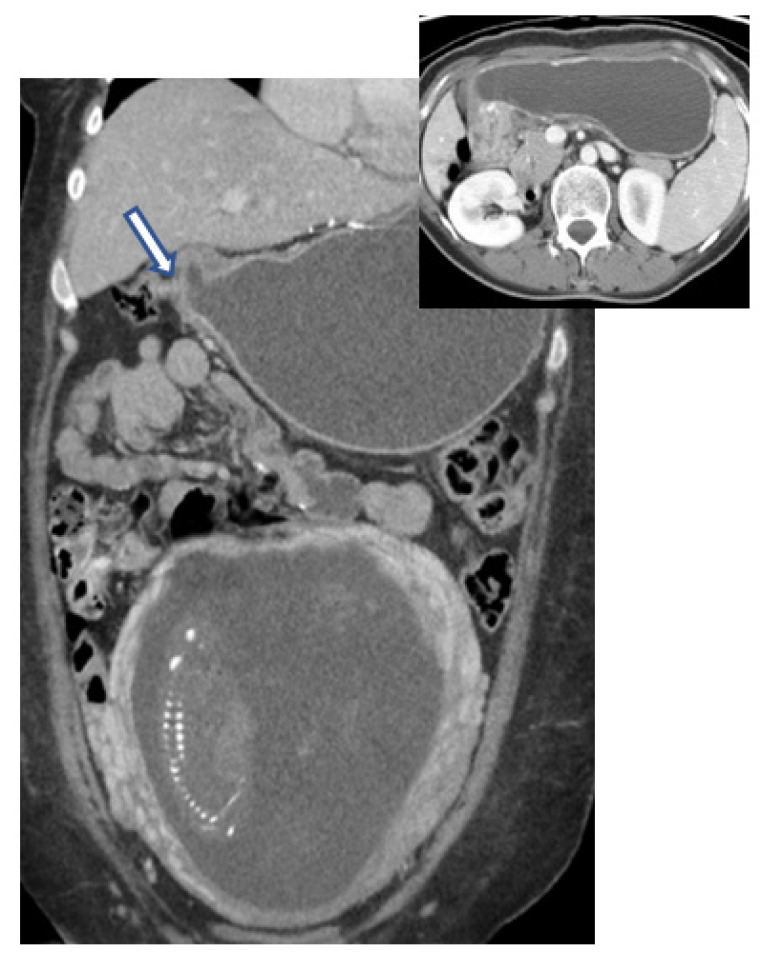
Computed tomography imaging of a pregnant woman (gestational age 16 weeks) with pyloric obstruction (arrow) caused by gastric adenocarcinoma.
